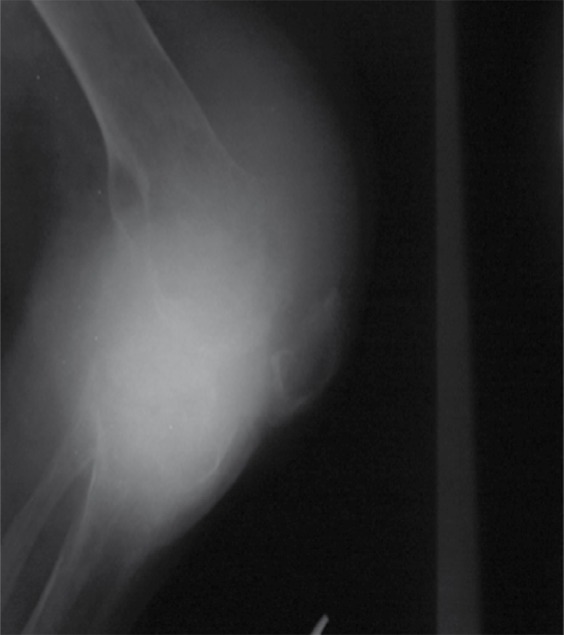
A 34-Year-Old Man Presenting With a Hot and Painful Right Knee With Severe Soft Tissue Swelling. Plain radiography shows multiple lytic, well-defined, narrow transitional zone bone lesions in the distal of the femur and proximal of the tibia with patellar involvement, juxta-articular osteoporosis, soft tissue density in the suprapatellar pouch.
